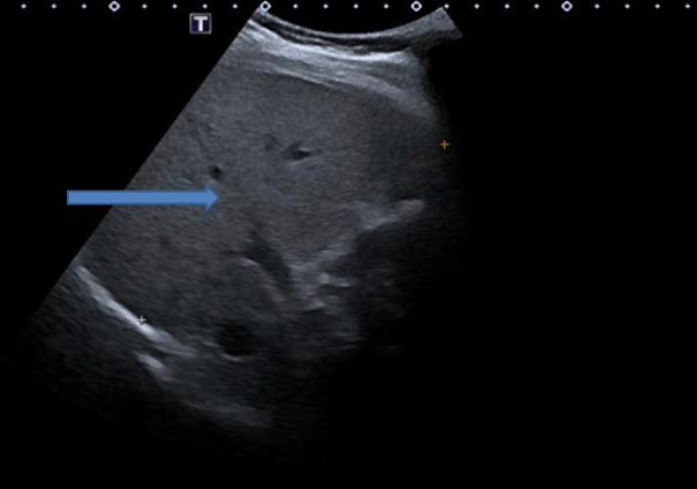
Ultrasonography of the abdomen revealed enlarged size of the liver with increased nodularity (blue arrow)
